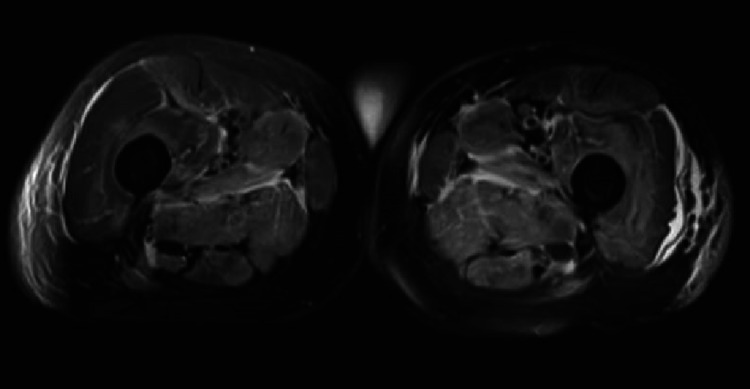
MRI of the bilateral lower extremities (quadriceps muscles) with and without intravenous contrast. There is enhancing feathery T2 hyperintense signal in the musculature of the proximal thighs bilaterally. Involvement is most pronounced in the adductor, obturator, and tensor fascia lata musculature with patchy involvement of the visualized proximal hamstrings and quadriceps musculature.
